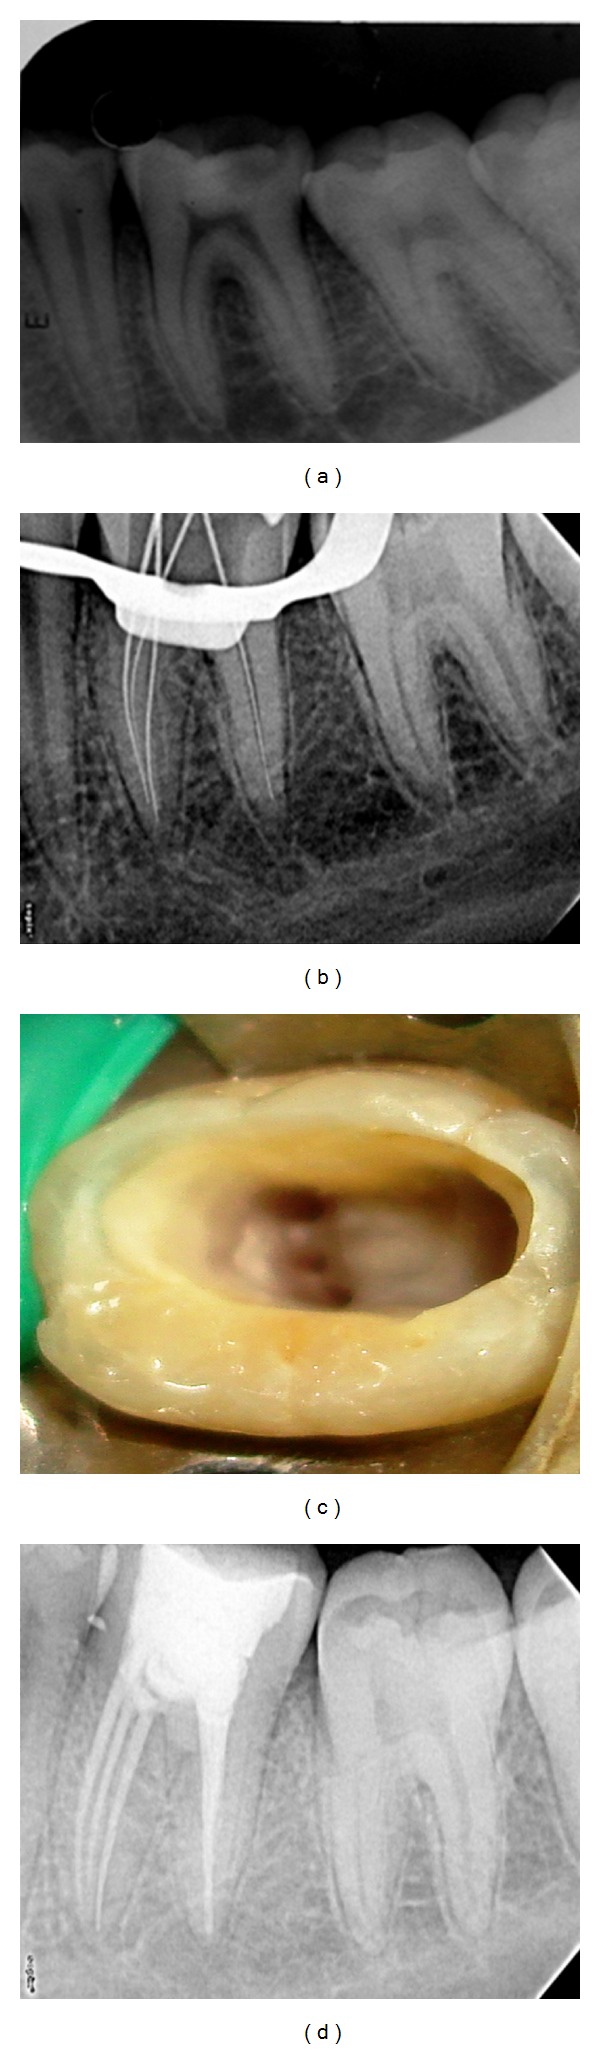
(a) Preoperative radiograph. (b) Working length. (c) Clinical image. (d) Obturation.
